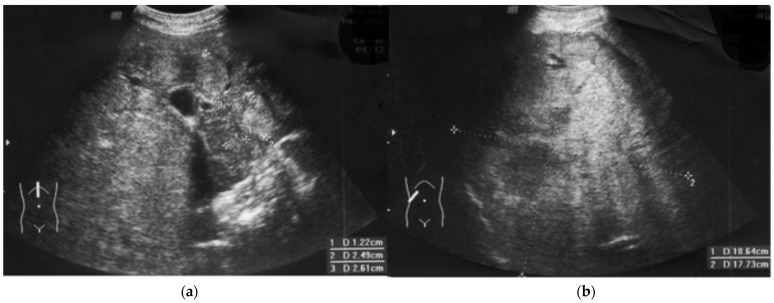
Fulminant hepatocellular carcinoma HCC progression in a patient with immunodeficiency. Regularly (every 3 months) performed ultrasound examinations (liver, spleen, lymph nodes) did not show any changes in October, but the ultrasound image showed heterogenous nodules (January), (a) that resemble nodular regenerative hyperplasia, typical for CVID [2]. The first blind liver biopsy (January) was non-diagnostic. Afterwards, a 110 mm tumor developed, and HCC was confirmed by second biopsy (April). Finally, fulminant growth up to 180 mm was observed despite lamivudine therapy, vaccination, normal ALT, and high anti-HBs level (b).
